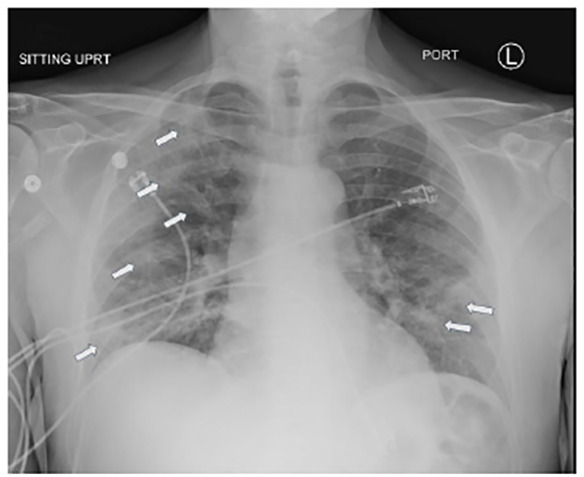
Radiograph demonstrating multifocal patchy ill-defined opacities (arrows) in bilateral lung fields, suggestive of atypical/viral pneumonia.
